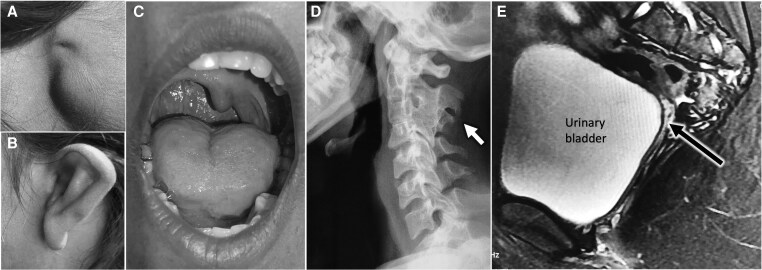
Clinical and imaging findings in case 1: (A) right anotia; (B) left abnormally shaped pinna; (C) asymmetry of the soft palate; (D) lateral radiograph of cervical spine showing fusion of cervical vertebrae C2, C3, and C4 (white arrow); and (E) sagittal section of magnetic resonance imaging pelvis showing rudimentary uterus (black arrow).
